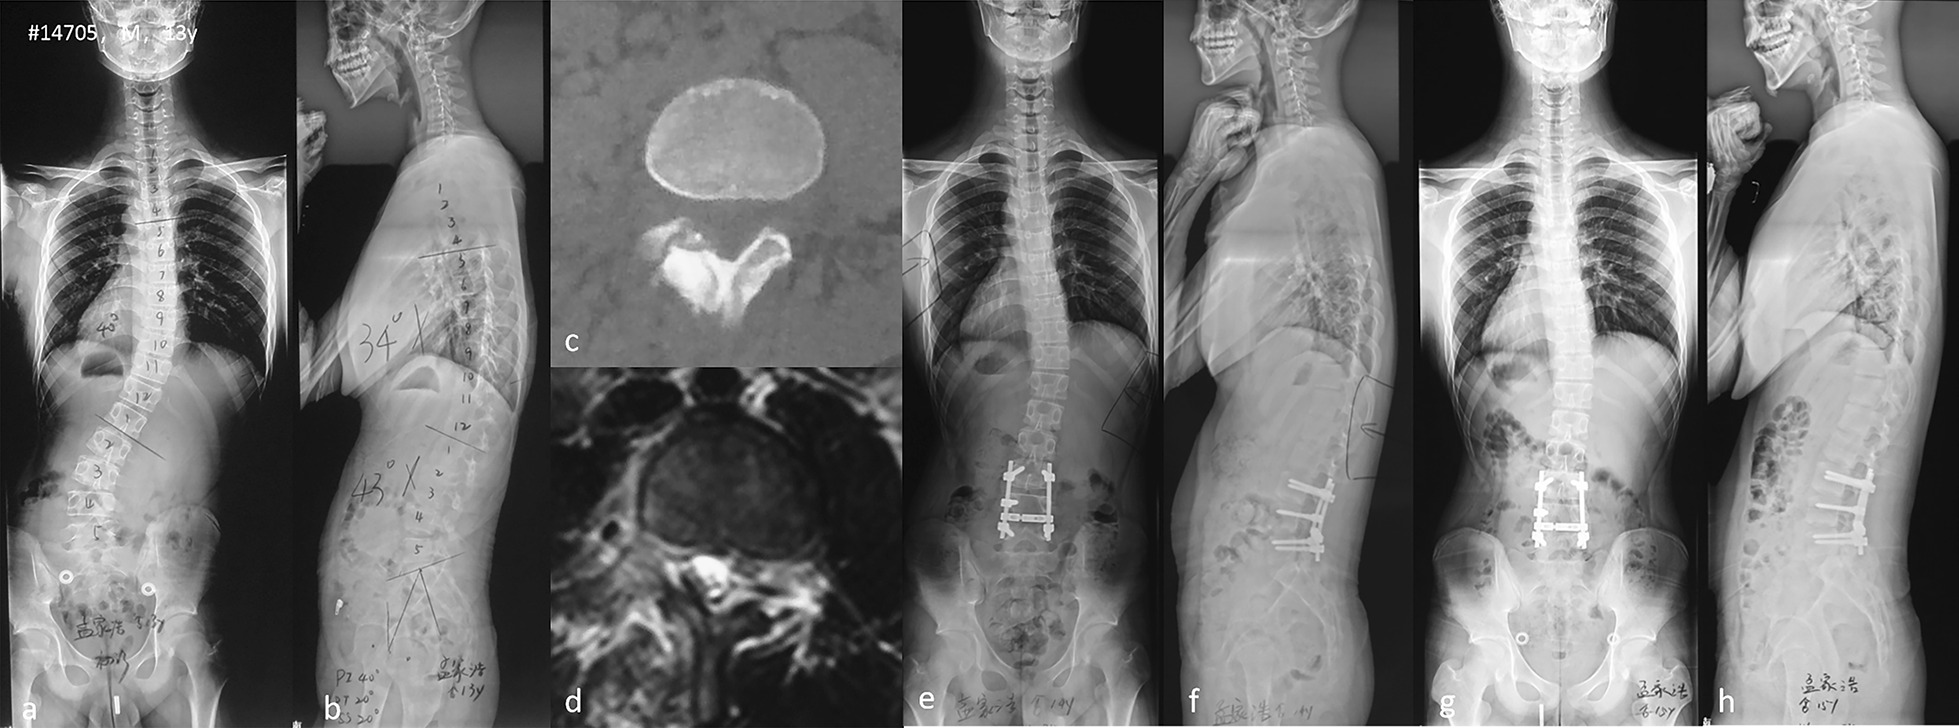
A 13-years-old male patient complained of back pain. X-rays demonstrated a 40° right thoracic scoliosis and a 28° left lumbar scoliosis (a) and normal sagittal alignment (b); CT demonstrated the nidus was located at right lamina (c), and MR showed edema around nidus (d); Thoracic scoliosis improved to 20°, and lumbar scoliosis improved to 8° at 3-month after surgery (e). Sagittal alignment was unchanged (f). Two-year follow-up X-rays showed that thoracic scoliosis was reduced to 16°, and lumbar scoliosis to 4° (g). No change of sagittal alignment was observed (h)
